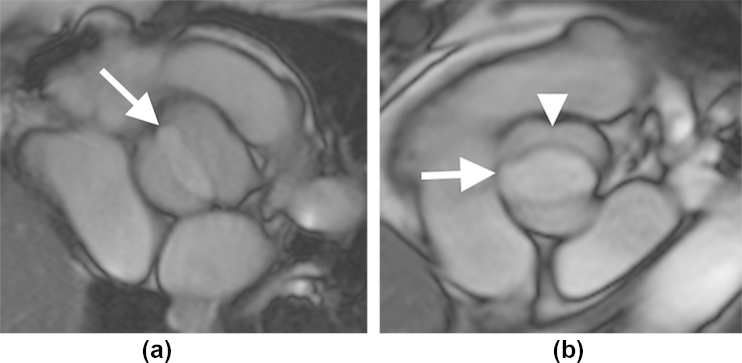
Systolic cine MRI of the BAV in two patients with TS. (a) Demonstrates a R-L BAV (arrow) and (b) demonstrates a R-L BAV (arrow) with raphe (arrowhead).
